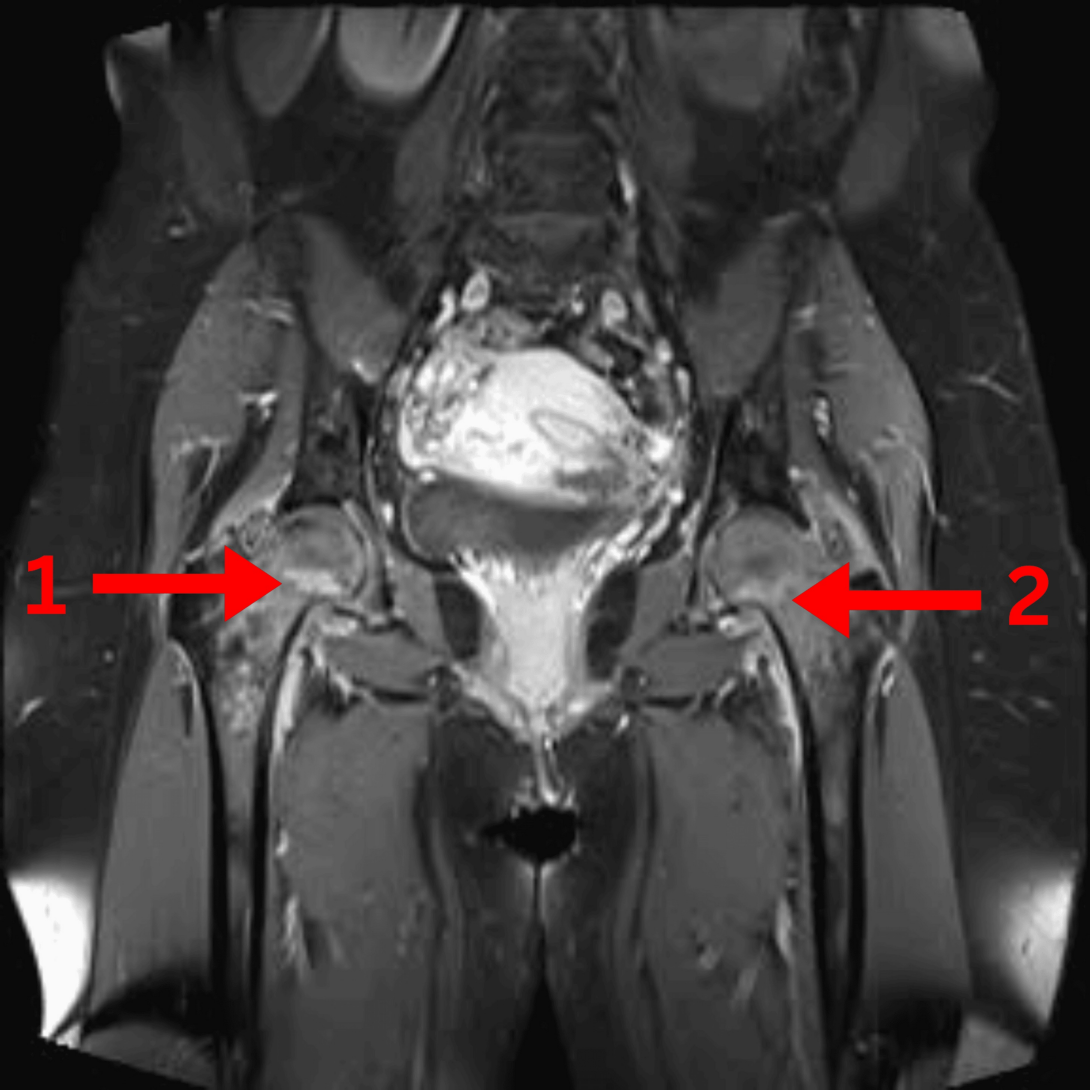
MRI of the hips with contrast showing (1) synovitis and (2) a fracture line MRI, magnetic resonance imaging.
